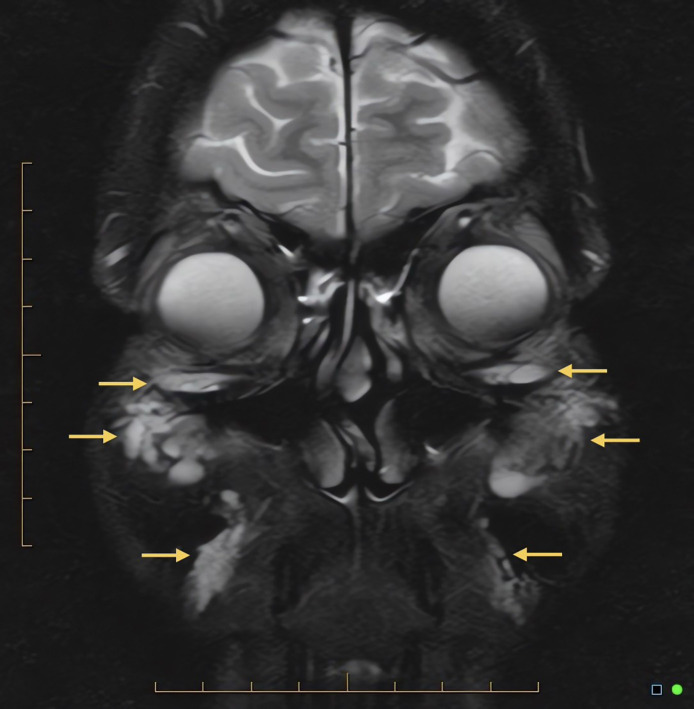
Coronal T_2_-weighted MRI demonstrating multiple T_2_-hyperintense foci (arrows) in the infraorbital, prezygomatic, deep cheek, and marionette regions. These signals are consistent with residual hyaluronic acid filler but may overlap with edema or other soft-tissue fluid.
